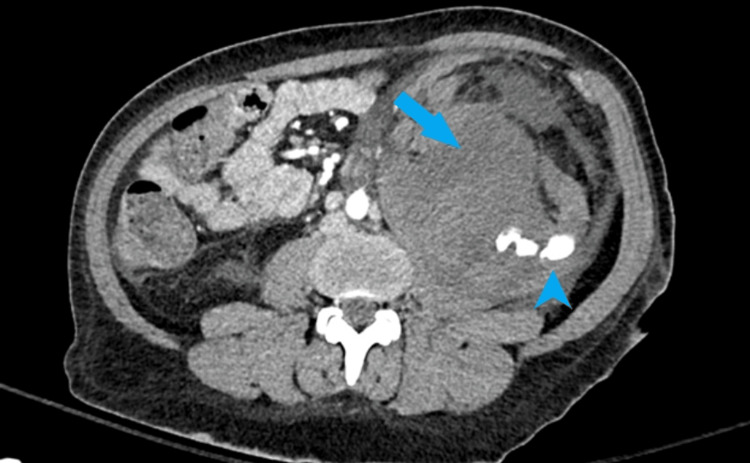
CT scan of the abdomen Active extravasation originating from the left kidney (arrowhead). The arrow shows a complex fluid representing hematoma formation in the kidney and pararenal space (measuring up to 14 cm).
